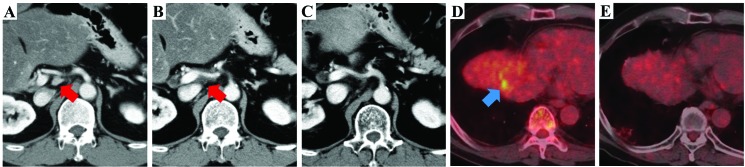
(A) Local lymph node metastases (red arrow) were detected along the CHA on CT scan. (B) Subsequent to switching from mFOLFOX6 treatment to TS-1, the recurrent tumor (red arrow) was identified again around the CHA. (C) Although the recurrent tumor disappeared subsequent to restarting treatment with mFOLFOX6, (D) the recurrent tumor (blue arrow) was revealed in the right subphrenic area on PET/CT. (E) The right subphrenic tumor disappeared following local radiation therapy and treatment with panitumumab and mFOLFOX6. CHA, common hepatic artery; CT, computed tomography; PET, positron emission tomography; mFOLFOX6, modified modified 5-fluorouracil, leucovorin and oxaliplatin regimen.
